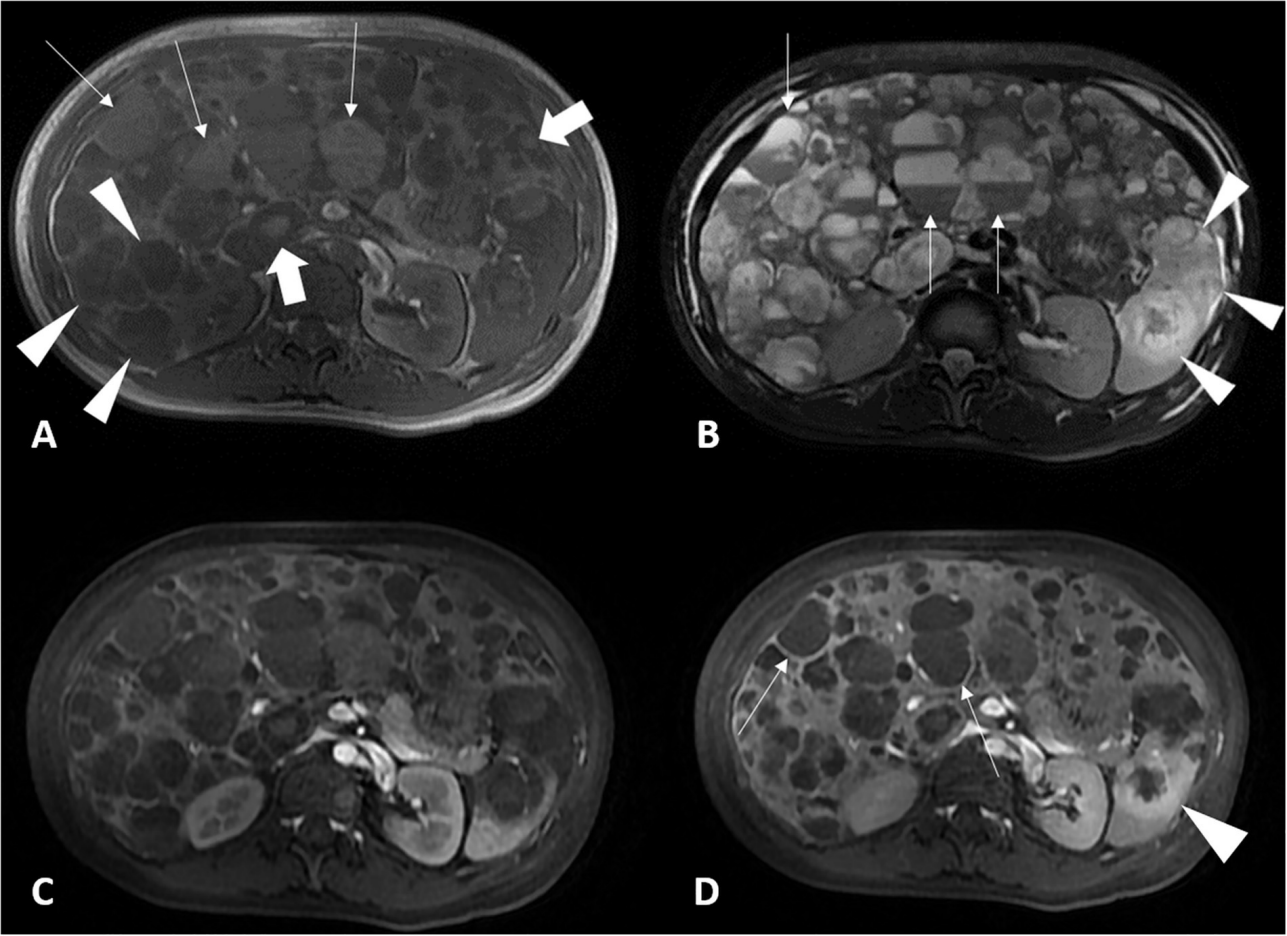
A 38-year-old women with primary hepatic angiosarcoma (case 2). (A) Transverse in-phase gradient-echo (TR/TE = 130/4.2) MR image shows multiple lesions display slight high (thin arrows), low (arrowheads) or both mixed signal intensity (thick arrows) at bilateral lobes of liver. (B) Transverse fat-suppressed T2-weighted fast spin-echo (TR/TE = 11428.6/91.312) MR image displays multiple lesions with mixed high and low signal intensity at bilateral lobes of liver. Some lesions show fluid-fluid levels (thin arrows). Splenic metastases are hyperintense on T2-weighted image (arrowheads), which are clearer than on in-phase MR image. (C) Transverse fat-suppressed T1-weighted (TR/TE = 100/1.8) post-contrast MR image on the arterial phase shows poor ring contrast enhancement in hepatic and splenic tumors. (D) Transverse fat-suppressed T1-weighted post-contrasted image on the portal phase displays ring enhancement at the peripheral zone of liver tumors (thin arrows) and splenic metastatic lesion (arrowhead).
